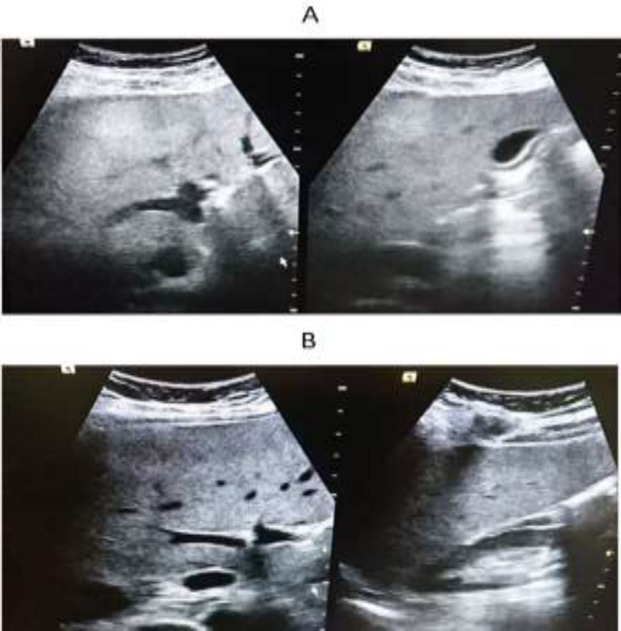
The initial liver ultrasound in a 56-year-old man shows fatty liver disease grade three. B: Repeated ultrasound after three months of vitamin D administration shows fatty liver disease grade one
